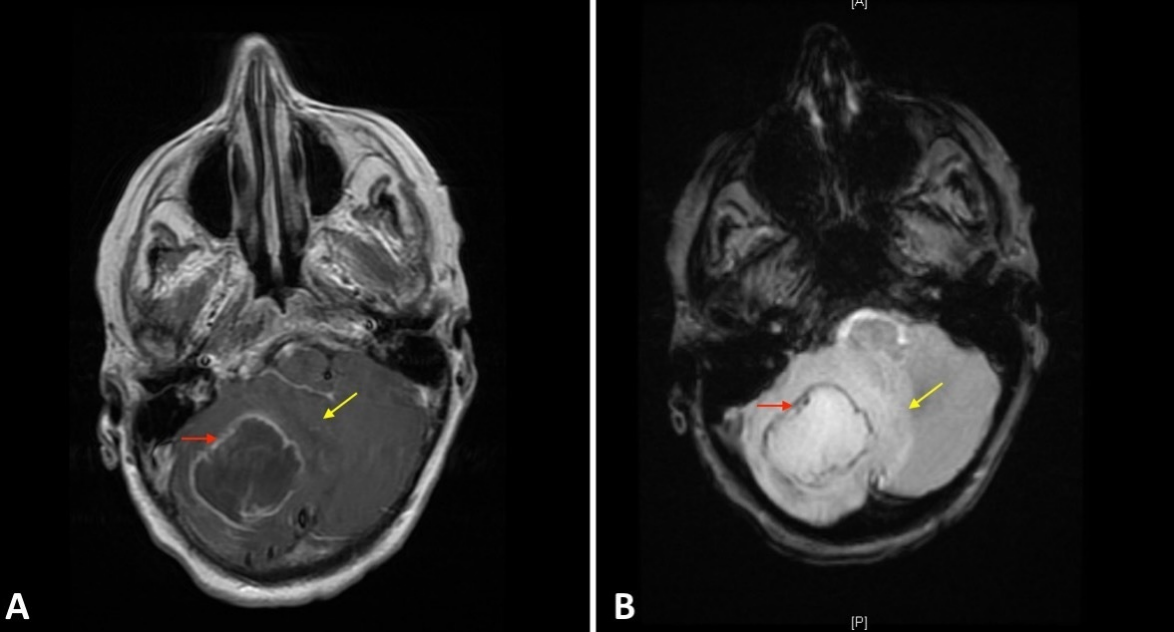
Imaging Findings A 4.6 cm abscess with rim-enhancing lesion in the right cerebellar hemisphere (red arrows) and extensive surrounding edema (yellow arrows) seen on (A) T1 post contrast and (B) diffusion weighted imaging (DWI).
